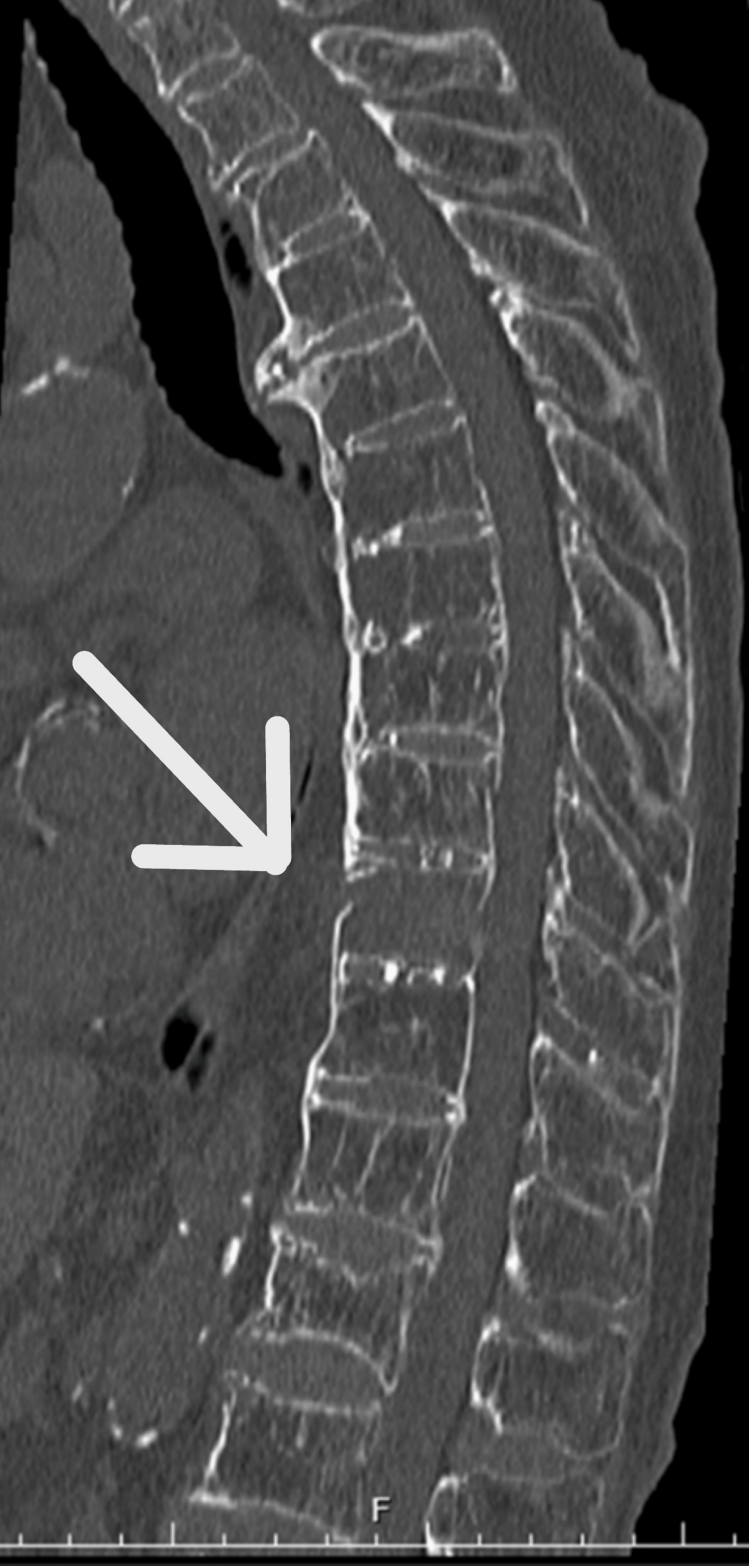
Sagittal reconstructed CT scan from the chest CT scan at the initial evaluation Discontinuity of the Th10 anterior vertebral wall was observed.
